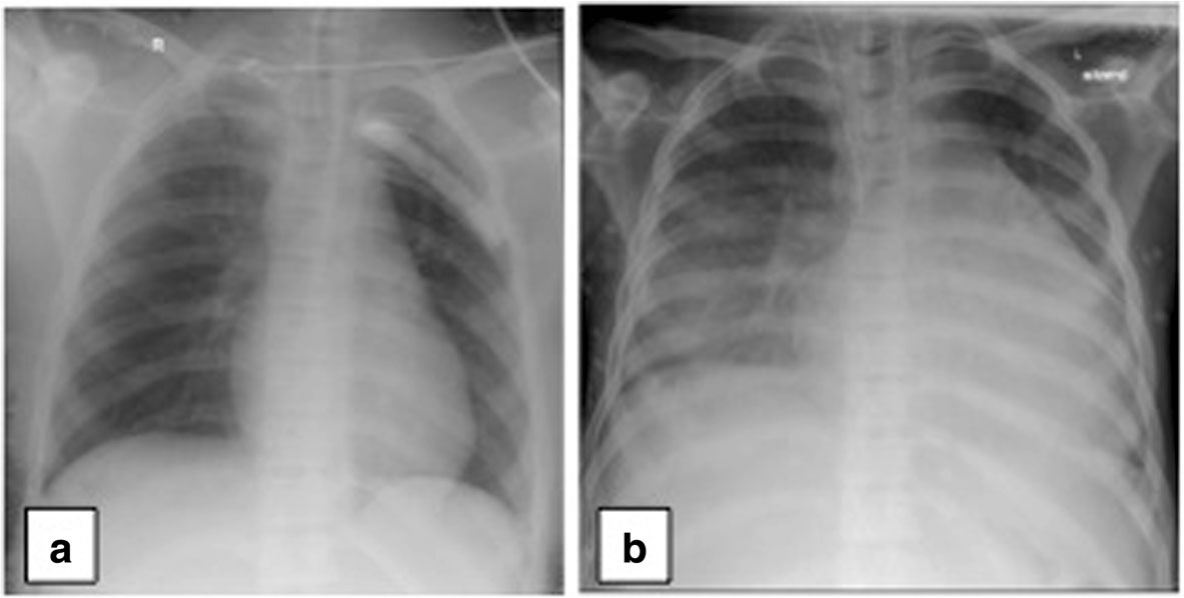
A chest X-ray showed an increase of the cardiothoracic ratio from 0.50 (a) at the time of admission to 0.63 (b) at the 10th week postburn
